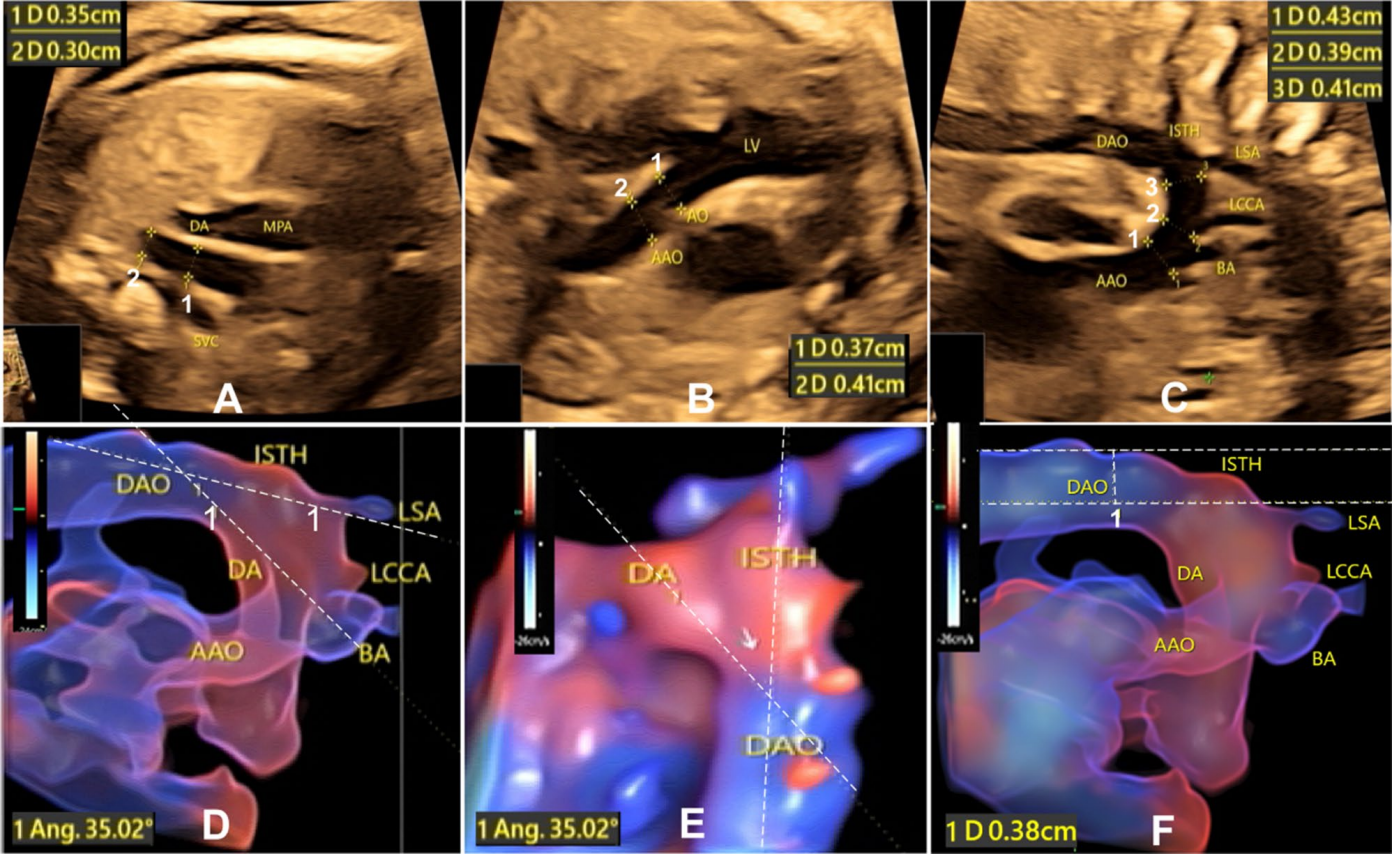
Parameters measured on ultrasound images. A. The diameters of the transverse aorta (1) and the aortic isthmus (2) were measured on the 3 vessel trachea view, with the transverse aorta diameter of 0.35 cm and the isthmus diameter of 0.30 cm. B. The diameter of the aorta (AO) at the aortic valve annulus and the ascending aorta (AAO) was measured at the left ventricular outflow tract section, with the AO diameter (1) of 0.37 cm and the AAO diameter of 0.41 cm. C. The diameter of the aortic arch was measured at three locations: arch 1 before the brachiocephalic artery (BA), arch 2 before the left common carotid artery (LCCA) and arch 3 before the left subclavian artery (LSA). D. The ductus arteriosus (DA)-isthmus angle was measured on the HD Live Flow image on the sagittal view, with the DA-Isthmus angle of 35.02°. E. The DA-isthmus angle was measured on the HD Live Flow image viewed from the bird’s eye’s view, with the DA-Isthmus angle of 35.02°. F. The displacement (or distance) from the LSA origin to the DAO outer edge were measured on the 3D STICK-HD Live Flow image. MPA, main pulmonary artery; SVC, superior vena cava; ISTH, aortic isthmus
